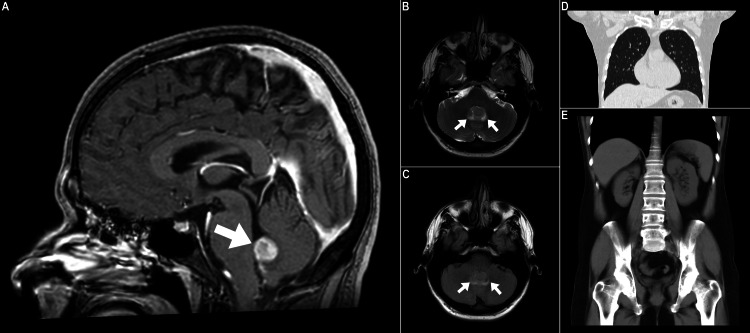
Baseline brain MRI. (A) Initial sagittal T1-weighted image performed post-gadolinium contrast medium administration, (B) T2-weighted axial view, and (C) fluid attenuation inversion recovery (FLAIR) axial brain MRI sequences. The study revealed a fourth ventricular mass measuring 1.5 cm in maximum dimensions (arrows), and demonstrating contrast enhancement with intermediate signal on T2-weighted and FLAIR sequences. Notably, there is very subtle edema surrounding the fourth ventricle. (D, E) Coronal chest, abdomen, and pelvic CT views were unremarkable for any concerning extracranial neoplastic lesions.
